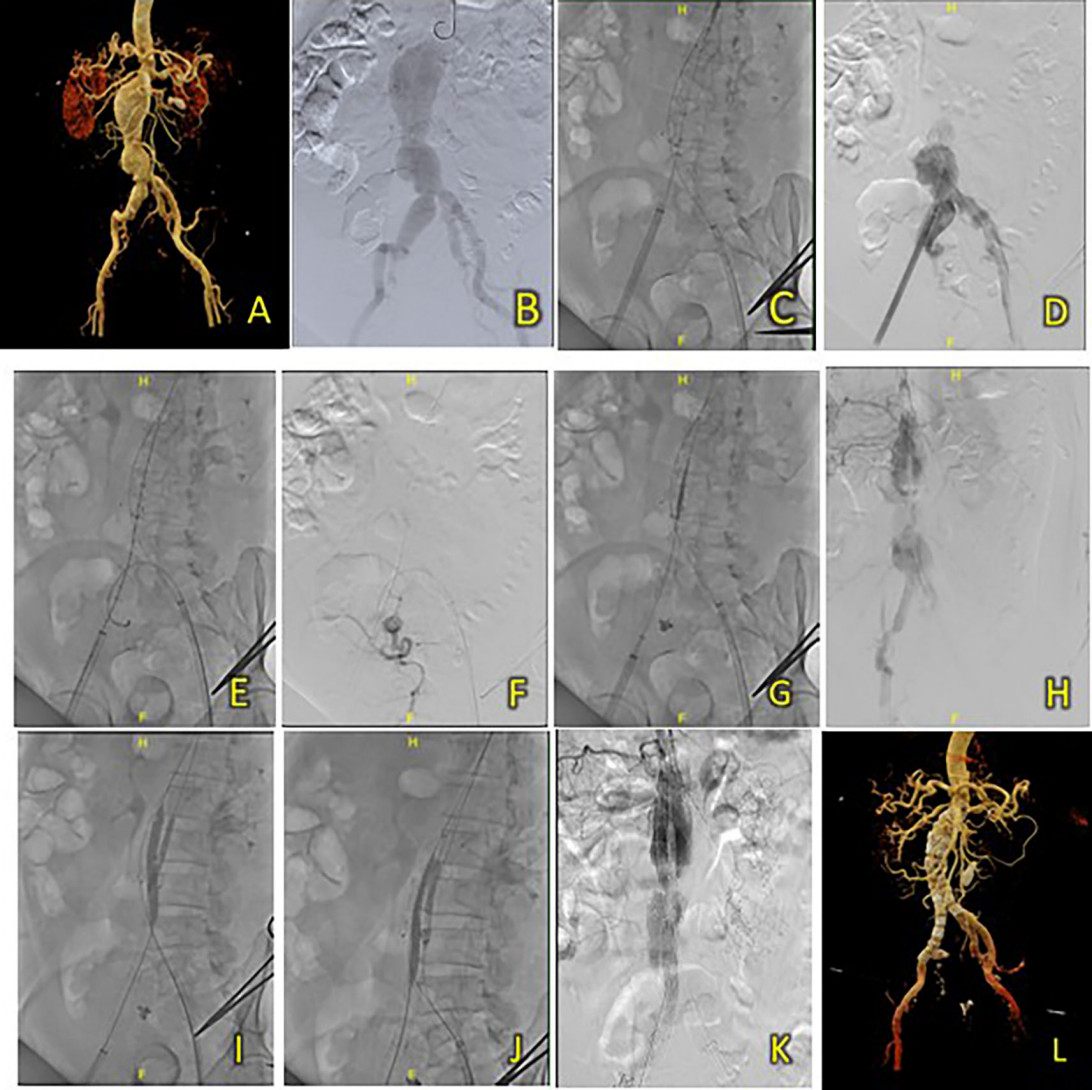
T&K B-EVAR on Patient 3. (A) Pre-procedure CT scan of the 3
